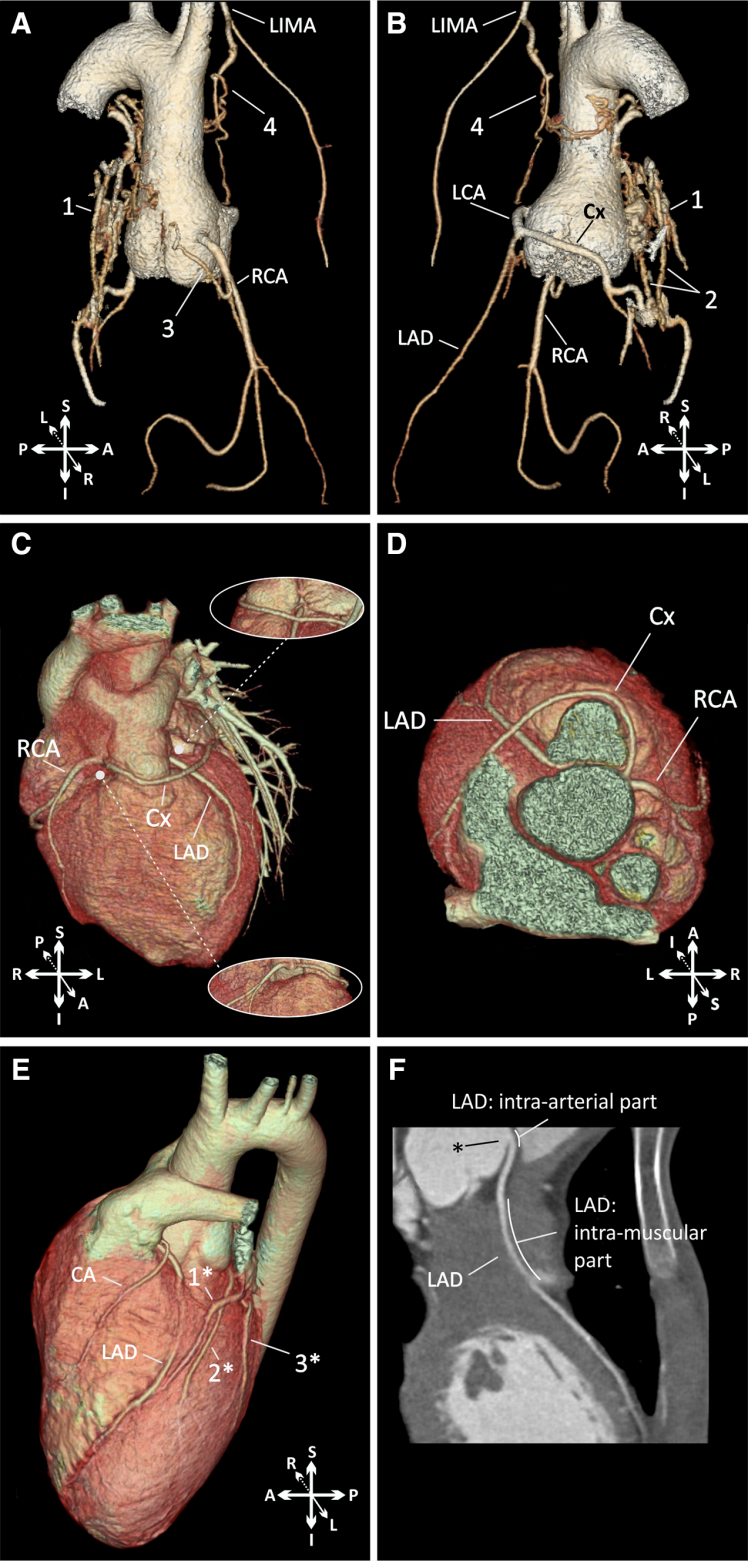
Examples of coronary computed tomography angiograms. a, b Volume-rendered 3D reconstructions of patient with coronary artery fistulas. *1* retroaortic conglomerate of arterial vessels connected with the RCA, Cx, LIMA, RPA, and LPA; *2* coronary fistulas between the Cx and right and left pulmonary arteries; *3* coronary fistula between the RCA and right pulmonary artery; and *4* fistula between the LIMA and the retroaortic conglomerate of arterial vessels. c, d Patient with a coronary anomaly (volume-rendered 3D reconstructions, description in text) and acute proximal angulation of the left anterior descending coronary artery (changes related to the proximal intra-vessel tangential course). e, f Patient with a coronary anomaly (Cx branching from the RCA) and long intramuscular course of the left anterior descending artery. *1 great cardiac vein; *2 accessory left anterior descending artery; *3 ramus intermedius artery; *LCA* left coronary artery, *Cx* circumflex coronary artery, *LAD* left anterior descending artery, *RCA* right coronary artery, *LIMA* left internal mammary artery, *CA* conal artery2D curved multiplanar reconstructions of the main coronary arteries (Fig. 2F);Diameters of the aortic valve, aortic sinus, sinotubular junction, and the ascending and descending aorta;Great vessel setup (the angle between the line connecting the middle of the sternum and vertebra and the line connecting the middle of the aortic and pulmonary valves in the short axis—Fig. 3);
